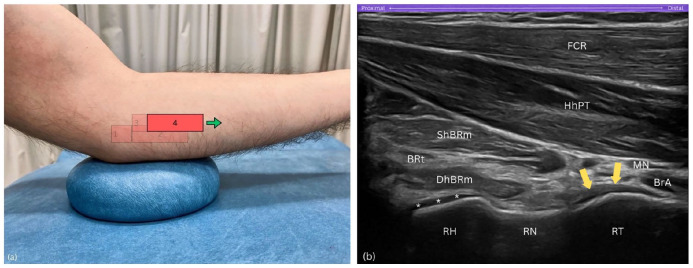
Step 4. (a) Further distal slide of the probe in a true horizontal direction. (b) Radial arc: view includes the radial head (RH) as a hill on the left, radial neck (RN) as a valley in the centre, and radial tuberosity (RT) as a hill on the right side of the sonogram. BRt, brachialis tendon; BrA, brachial artery; DhBRm, deep head of brachialis muscle; FCR, flexor carpi radialis; HhPT; humeral head of pronator teres; MN, median nerve; RH, radial head; RN, radial neck; RT, radial tuberosity; ShBRt, superficial head of brachialis muscle; asterisks (*), hyaline cartilage; yellow arrows, distal biceps brachii tendon.
